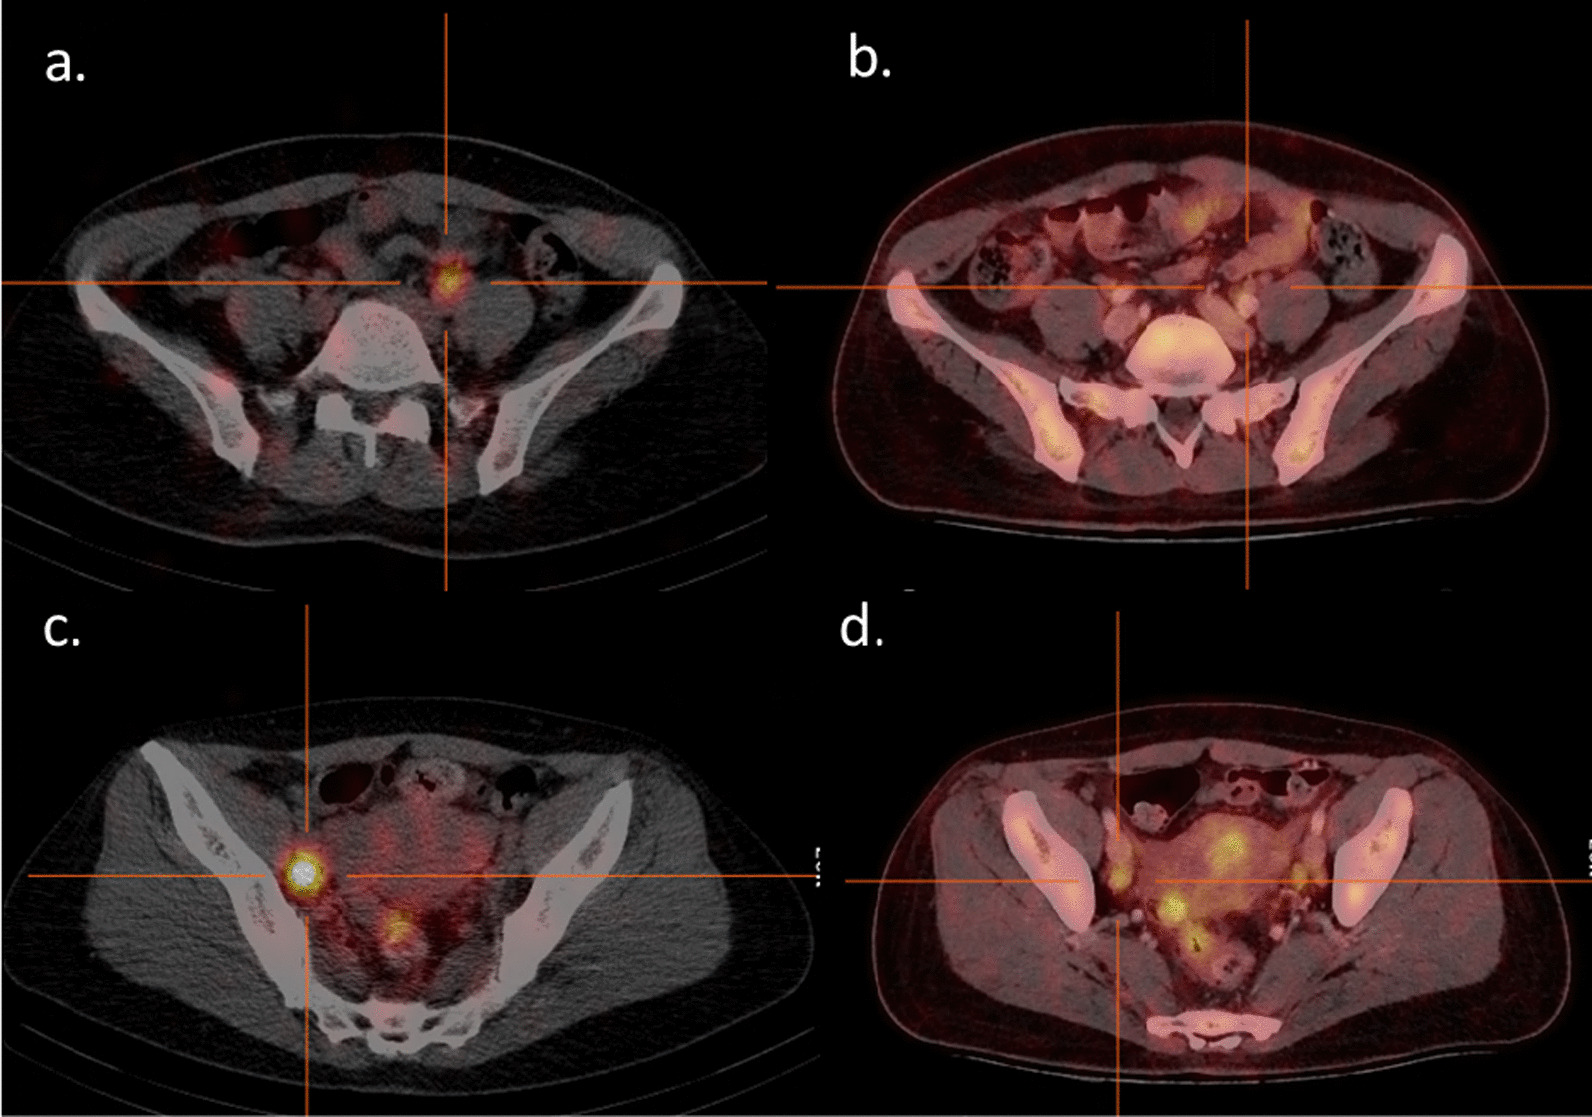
Lymphatic map (fused axial SPECT/CT images 24 h post-injection of [^99m^Tc]Tc-nanocolloid) and [^18^F]FDG-PET/CT images (fused axial images 1 h post-injection of [^18^F]FDG) of a patient with stage rIIIC1 squamous cell carcinoma. Upper row: lymph node at the level of the left common iliac vessel, not irradiated as it was not suspicious on MRI or [^18^F]FDG-PET/CT imaging. a Lymphatic map: visualized b [^18^F]FDG-PET/CT: no [^18^F]FDG uptake, short axis < 1 cm. Lower row: lymph node at the level of the left external iliac vessel, irradiated as it was suspicious on [^18^F]FDG-PET/CT imaging. c Lymphatic map: visualized. d [^18^F]FDG-PET/CT: pathological [^18^F]FDG uptake and 1.3 cm short axis
